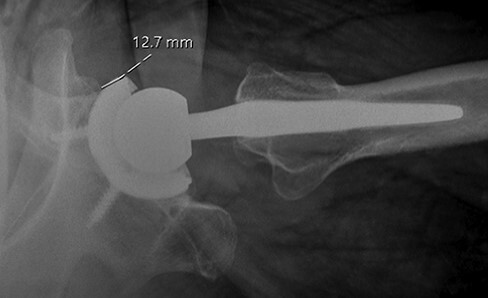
Cross-table lateral radiograph of the right hip measuring the amount of acetabular component prominence from the anterior acetabular rim.
